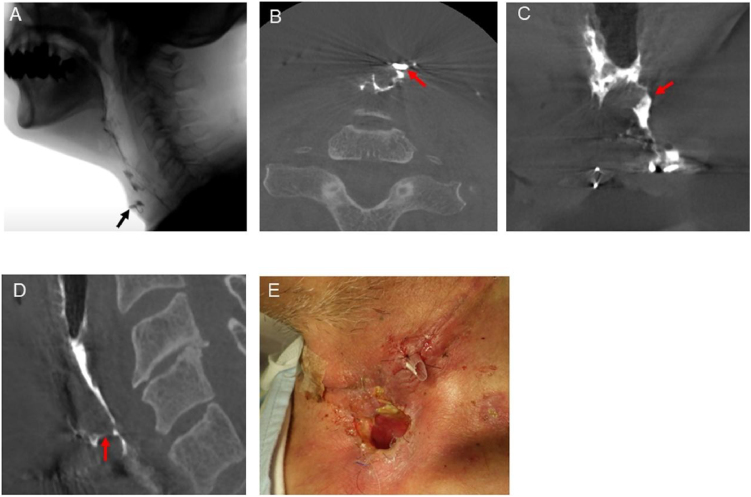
Videofluoroscopy and contrast CBCT findings of Case 2. A, Videofluoroscopy showing the leakage of contrast fluid. The black arrow indicates the leakage. B, Axial image of contrast CBCT showing the accurate location of leakage on the cervical side. C, Coronal image of contrast CBCT showing the accurate location of leakage on the pharyngeal side, which was located not at the midline, but on the left side. D, Sagittal image of contrast CBCT detected another leakage, which could not be observed by videofluoroscopy. The red arrows indicate the leakage. E, Appropriate surgical treatment was performed.
